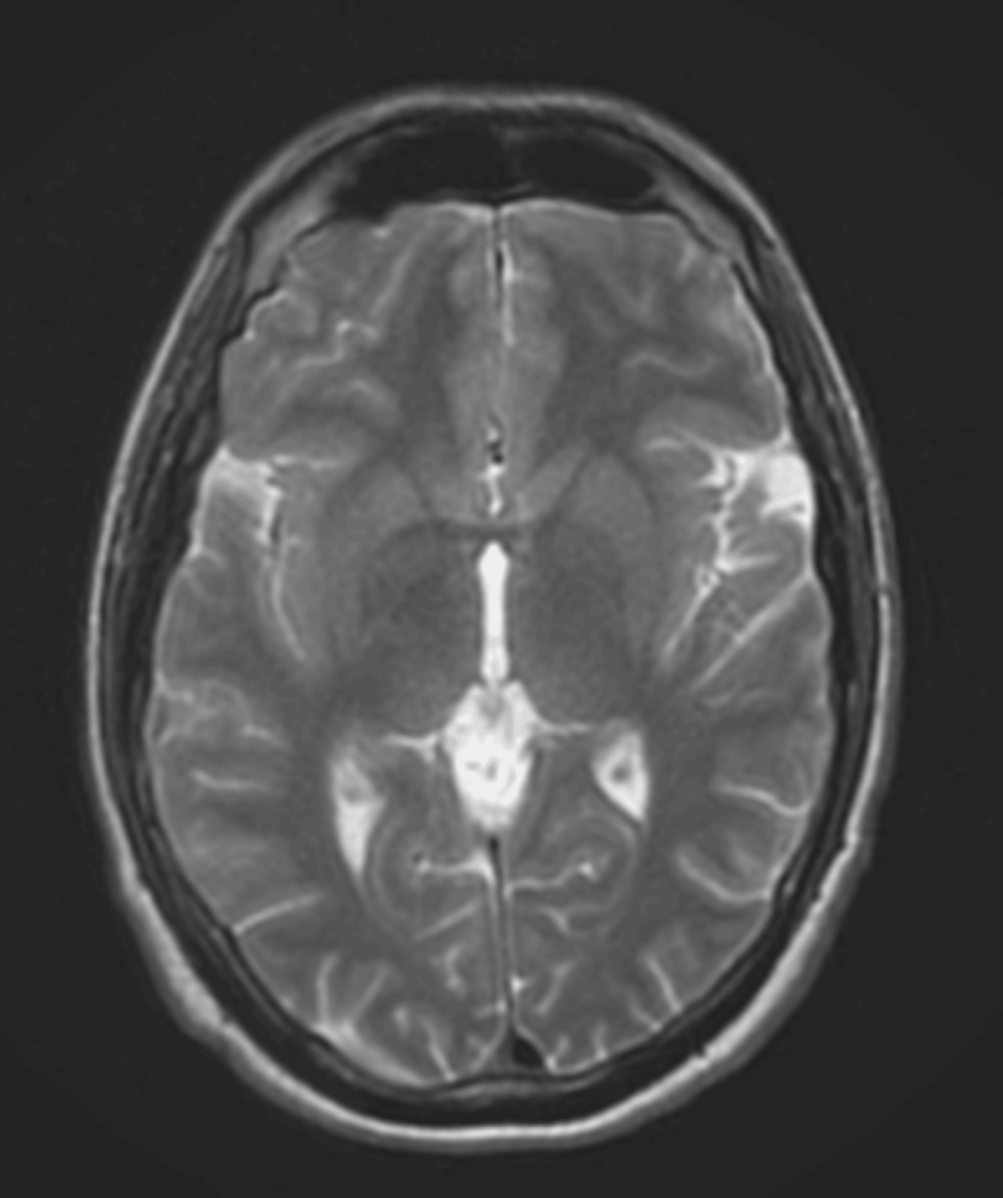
T2-weighted MRI of the brain with and without contrast (11/14/22) Impression: No significant intracranial process. No acute abnormality. Clinical examination: 24-year-old male with meningitis and elevated intracranial pressure
